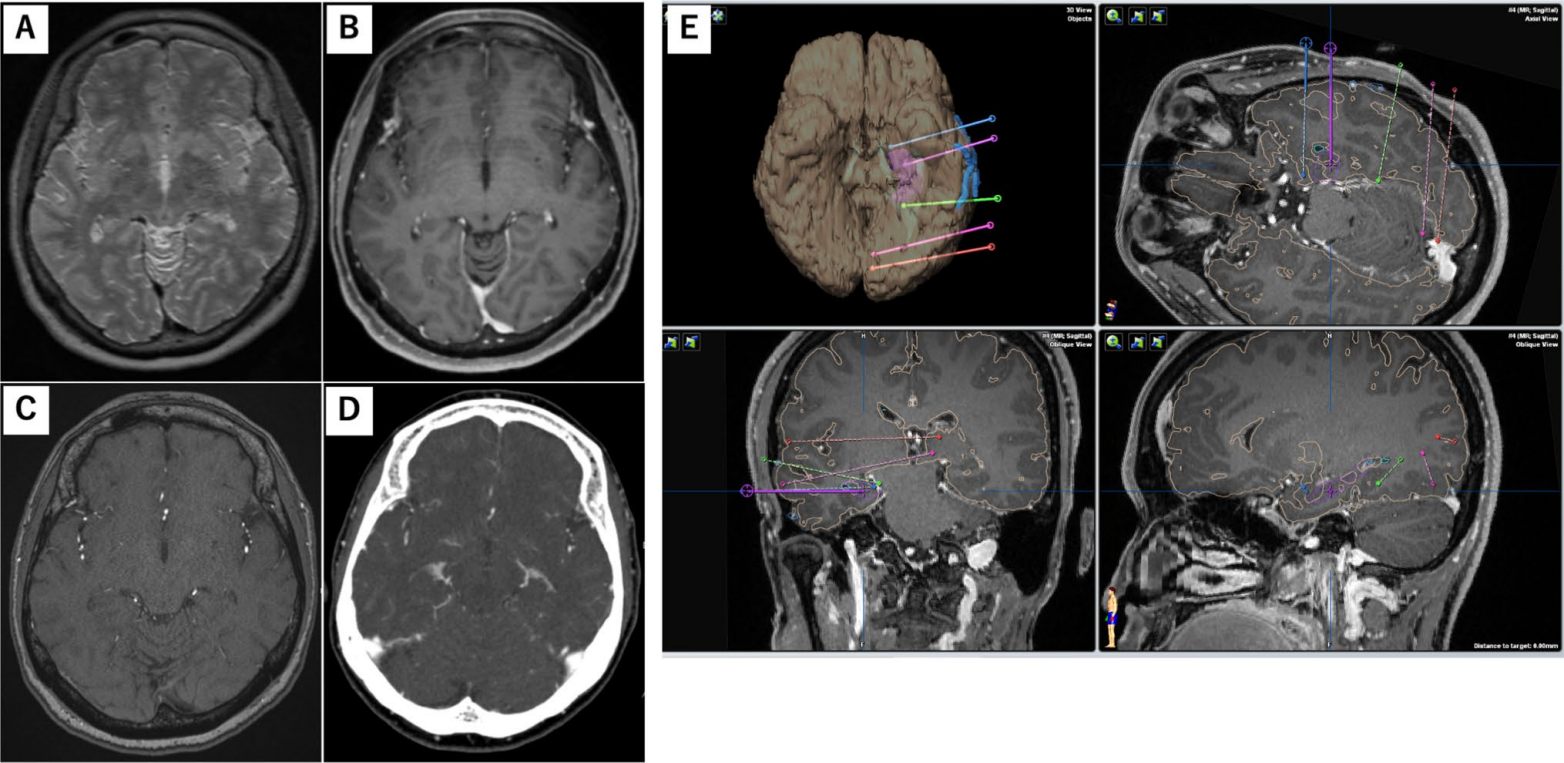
Preoperative planning of SEEG with T2 thin slice (A), enhanced T1 imaging (B), time of flight (C), and enhanced CT (D). SEEG planning and 3D reconstruction using iPlan (E)
